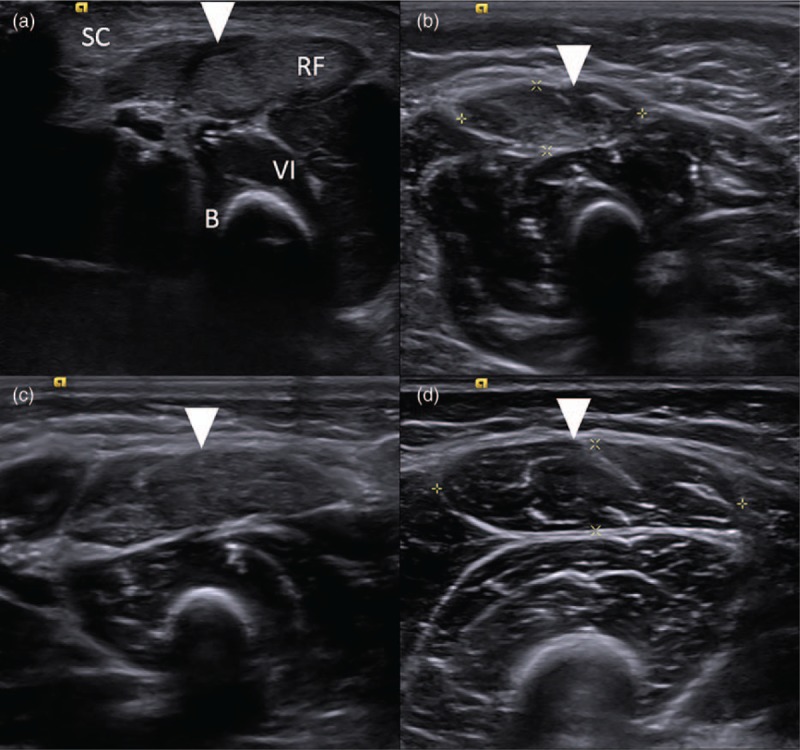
Representative muscle ultrasound images of the thigh with arrowheads pointing to the rectus femoris muscle in an (A) 10-day-old boy with infantile-onset Pompe disease classified as grade 3; (B) 46-day-old girl with infantile-onset Pompe disease classified as grade 2; (C) 30-day-old girl with pseudodeficiency mutation classified as grade 2; (D) 5-year-old girl identified as having late-onset Pompe disease by molecular genetic testing and grade 1 disease by muscle ultrasound examination. B = bone reflection, RF = rectus femoris muscle, SC = subcutaneous fat, VI = vastus intermedius muscle.
